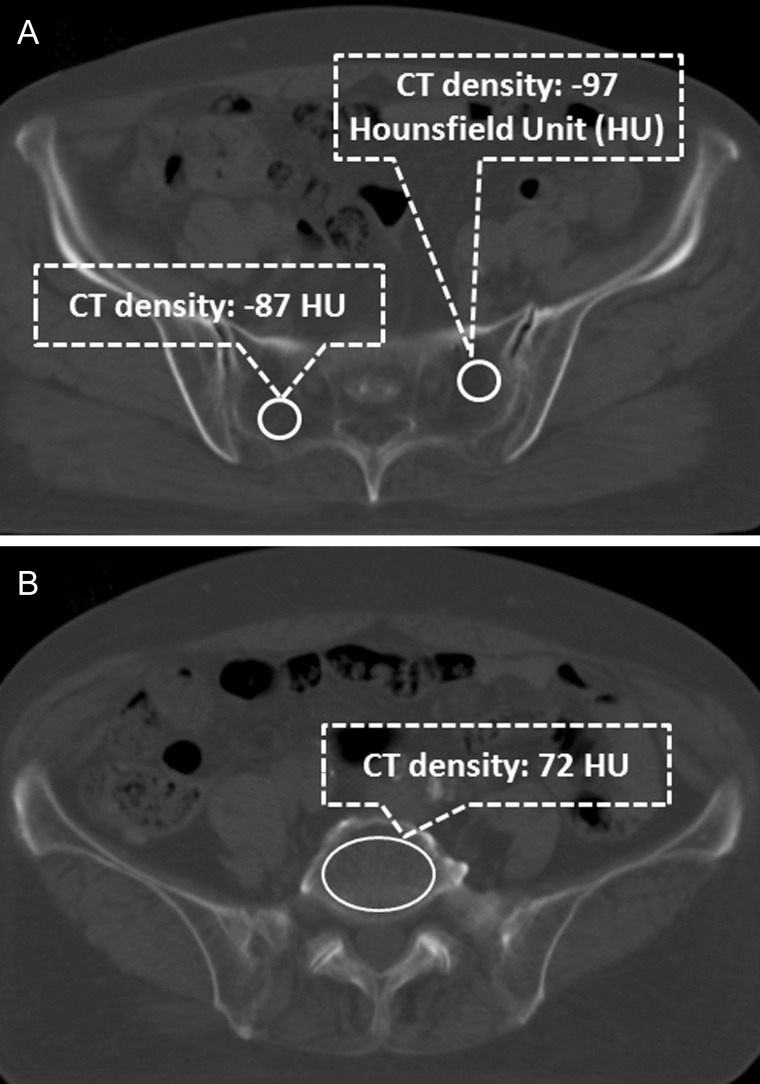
(A) Transaxial image of an RT-planning CT of a representative case. Area of visually lowest density of bone marrow in the right and left sacrum coincided with each other in this case. CT densitometry shows −87 and −97 HU on the right and left sacral bone marrow, respectively. (B) Transaxial image of a RT-planning CT of the same case as Fig. 1A. L5 lumbar vertebra shows the most homogenous bone marrow density on this slice. CT densitometry shows 72 HU on L5 lumbar vertebra.
